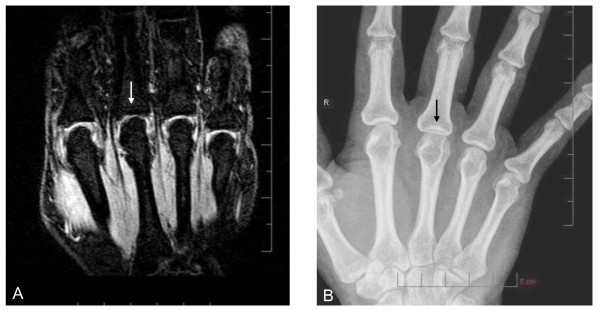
Joint-space narrowing (JSN) depiction in metacarpophalangeal (MCP) joint. Coronal, fat-suppressed, T_1_-weighted gradient-echo image of MCP joints 2 through 5 (A), and corresponding XR image (B) show grade-2.0 JSN of MCP 3 (arrow) but normal joint-space width of MCP 2, 4, and 5. The proximal interphalangeal joints are out of the plane of section on the MR image shown, but were visible on more-palmar sections of the scan (not shown).
